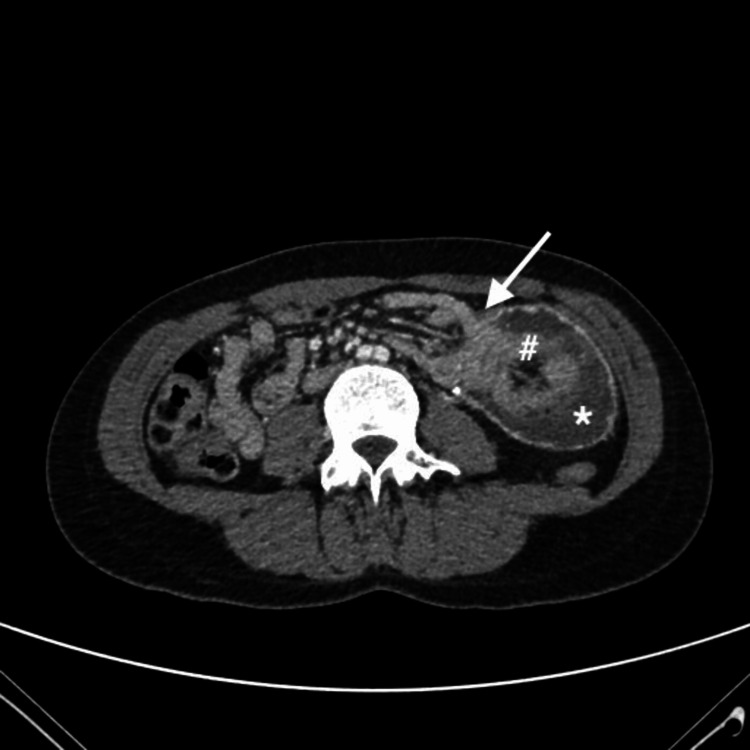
CT scan (axial plane). Findings consistent with jejunal loop intussusception at the gastrojejunal anastomosis. Arrow: the point of jejunal loop intussusception at the gastrojejunal anastomosis can be clearly identified; *: gastric chamber; #: jejunum.
